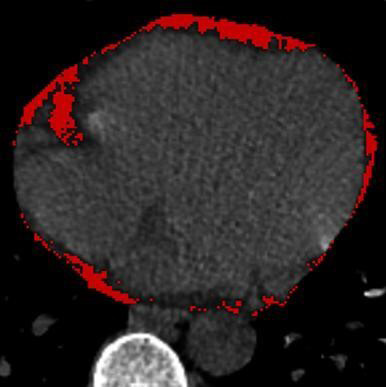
The measurement of EAT volume by non-contrast CT.
